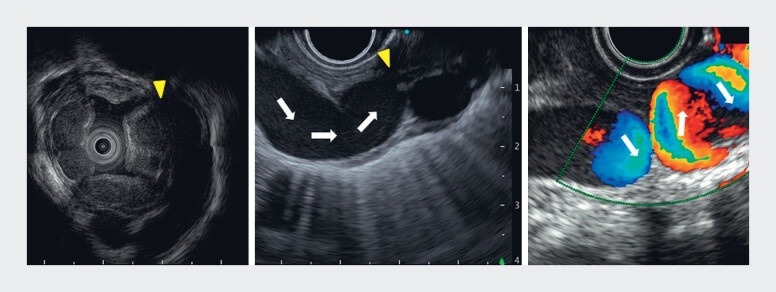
Endoscopic ultrasound images showing varices in which there were no palisade vessels (yellow arrowheads), with the pipeline varix flowing from left to right (white arrows).
